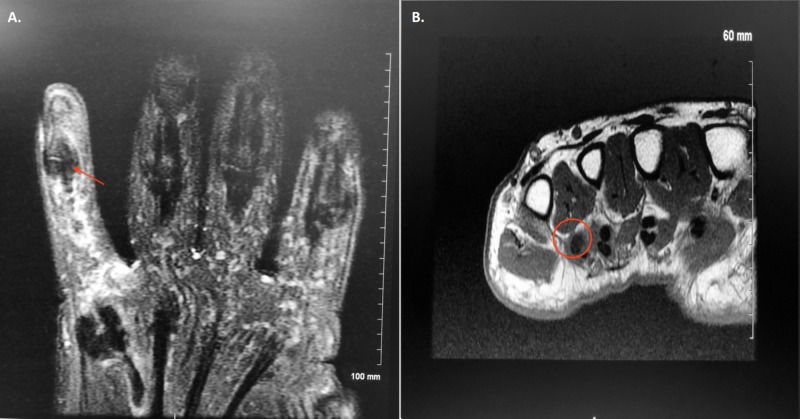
MRI scan of the right hand without contrast Impression: Complete tear involving the flexor digitorum profundus and superficialis tendons to the fifth digit located at the level of the mid-fifth metacarpal (red arrow). The proximal tendon stumps are not well visualized in the field-of-view. If further evaluation more proximally is clinically indicated, MRI of the wrist with the inclusion of the proximal metacarpals could be considered. There is some mild strand-like elevated transverse plane (T2) signal near the distal remaining tendons which may be due to edema or bruising (red circle). There is some strand-like elevated T2 signal in the subcutaneous tissues around the fifth digit which may be due to edema or bruising.
